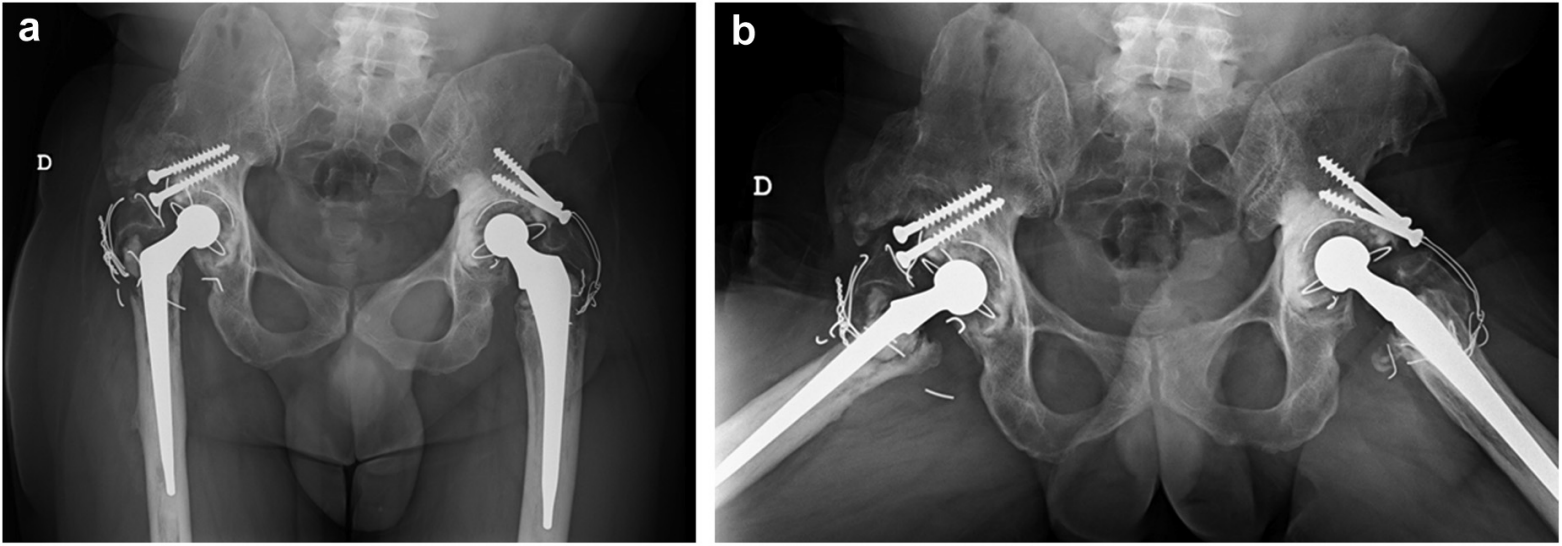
Anteroposterior (a) and frog-leg lateral (b) radiographs of the pelvis showing significant eccentric wear of the polyethylene mobile bearing and osteolysis around the right cup (DeLee and Charnley zone III) and the stem (Gruen zones 1 and 7). Some broken wires from a failed trochanteric fixation were also present.
